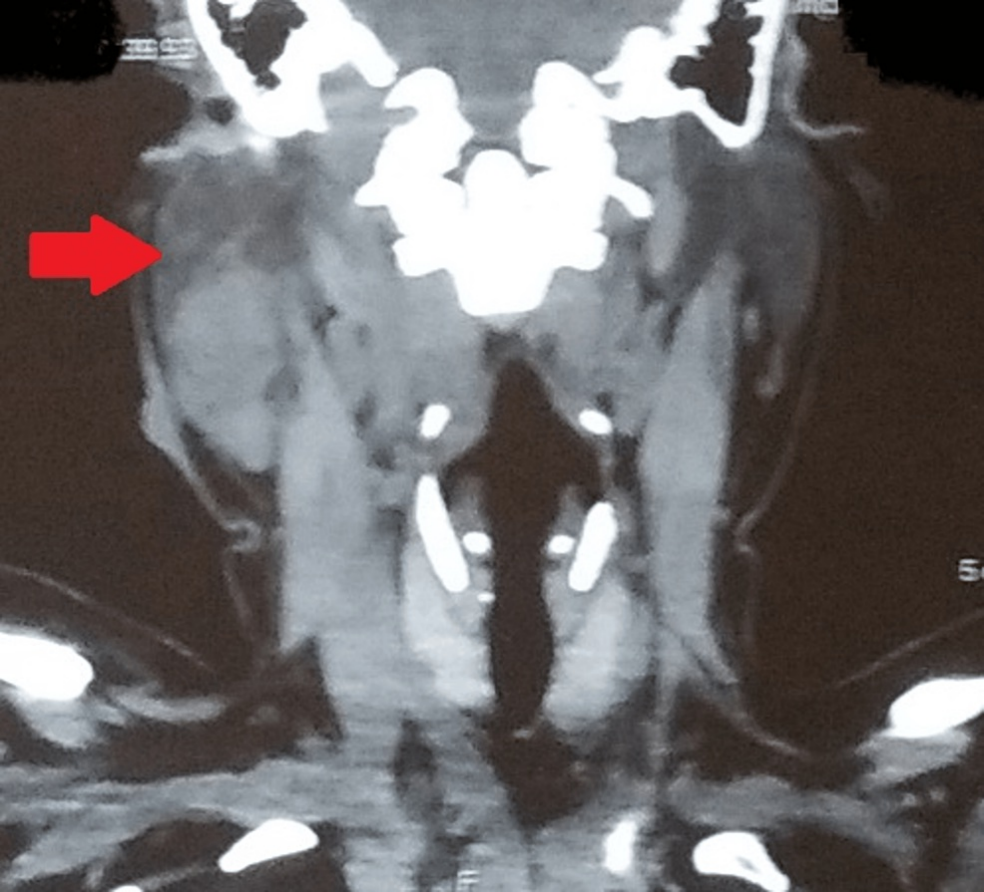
A multi-slice computed tomography (CT) of the neck (sagittal view) A multi-slice CT of the neck showing a bulky right parotid in its caudal aspect with a soft tissue density lesion within the parotid parenchyma closely abutting the sternocleidomastoid muscle
